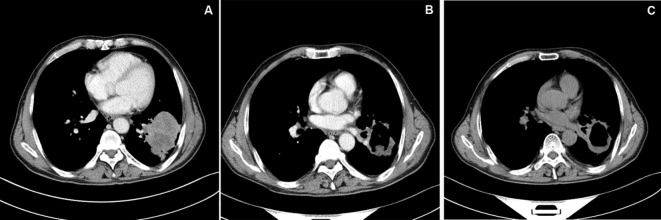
CT image of typical case. (A) Chest enhanced CT showed a mass shadow in the inferior lobe of left lung, about 5.8cm×6.7cm in size, with significant peripheral enhancement. (B) Chest enhanced CT examination at 1 month after DEB-BACE showed obvious necrosis of tumor lesion in the left lower lobe of lung, cavity formation in the lesion, reduced lesion area and no obvious enhancement. (C) Chest CT examination at 6 month after DEB-BACE showed obvious necrosis of tumor necrotic cavities in the linferior lobe of left lung was slightly larger than before, and the lesion area was further reduced.
